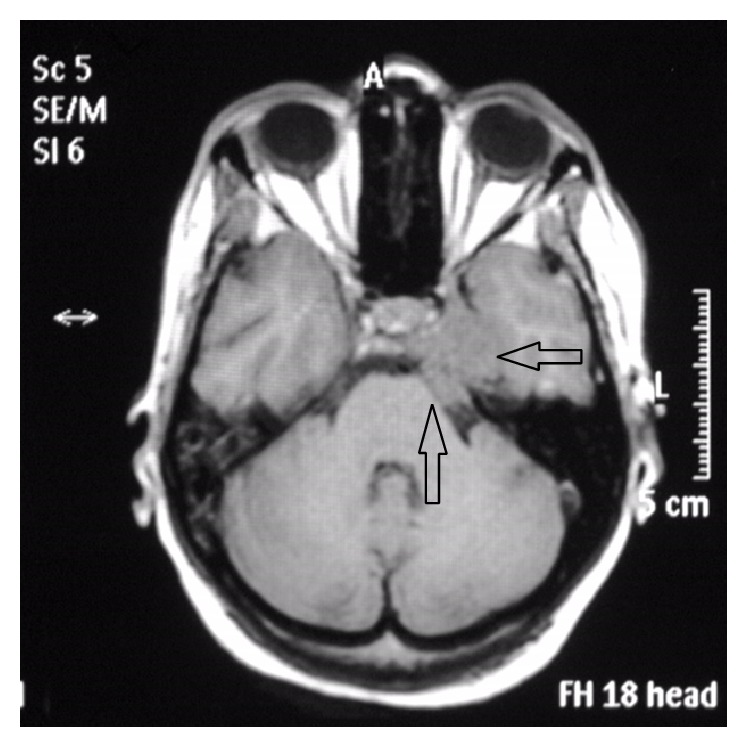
MRI precontrast showing altered signal intensity of the clivus, axial section that appears hyperintense.
